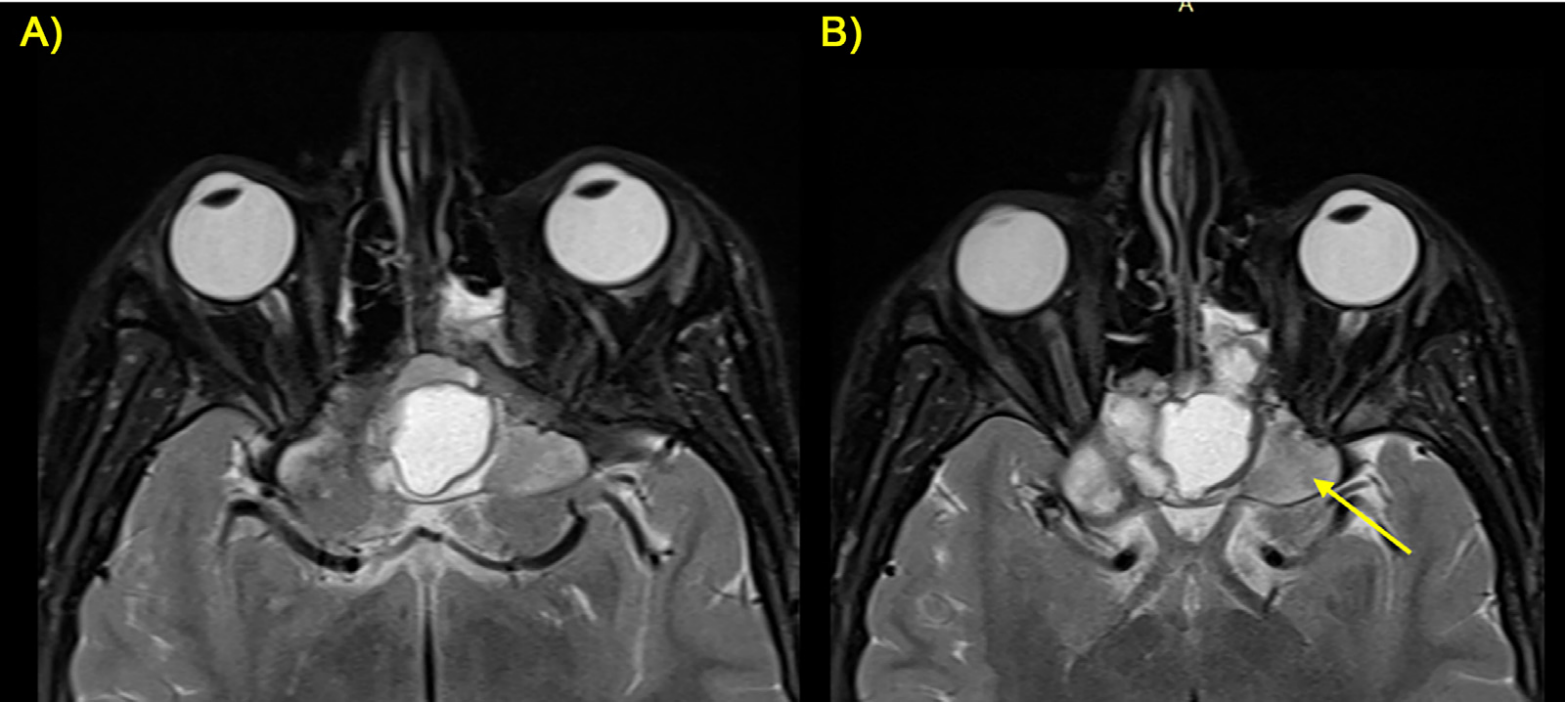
(A, B) Subsequential axial T2-w sequences performed on 3T MRI showed the tumor behavior with compression on the optic chiasm and the left optic nerve (yellow arrow).
